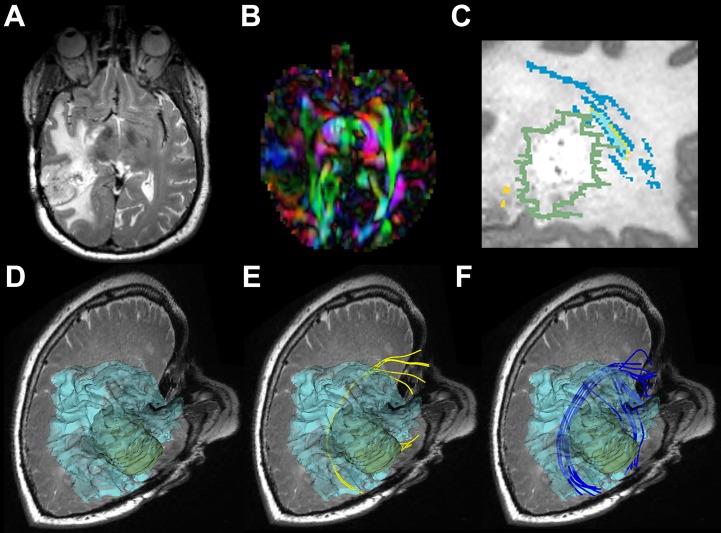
Images from P3 with a right temporal lesion. (a) T2-weighted image shows a temporal tumor with extensive edema around the lesion. (b) Directionally encoded color FA map shows reduced anisotropy in AF. (c) Magnified sagittal view of a T2-weighted image with overlaid label maps showing fibers reconstructed by the UKFt (yellow) and UKFt+FW (dark blue) methods and where they overlap (light blue) near the tumor (green outlined). (d) The reconstructed edematous area (transparent blue surface model) near the tumor (green model). (e) The AF using the UKFt method displayed in yellow. (f) The AF using the UKFt+FW method displayed in blue.
